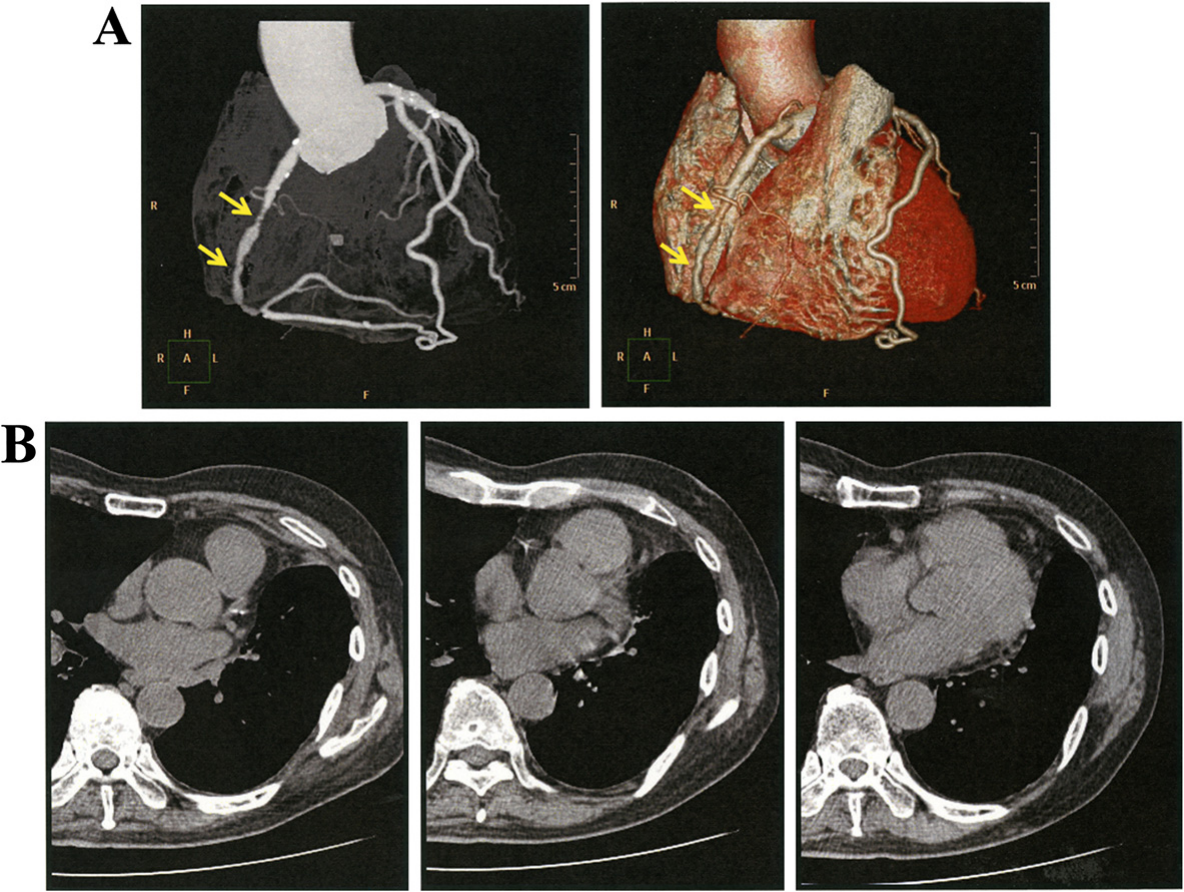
3D-CTA and conventional CT findings. Multiple areas with coronary stenosis (arrows) visualized by 3D-CTA (A) in an 82-year-old male patient with calcification grade 1 diagnosed by conventional CT (B).
